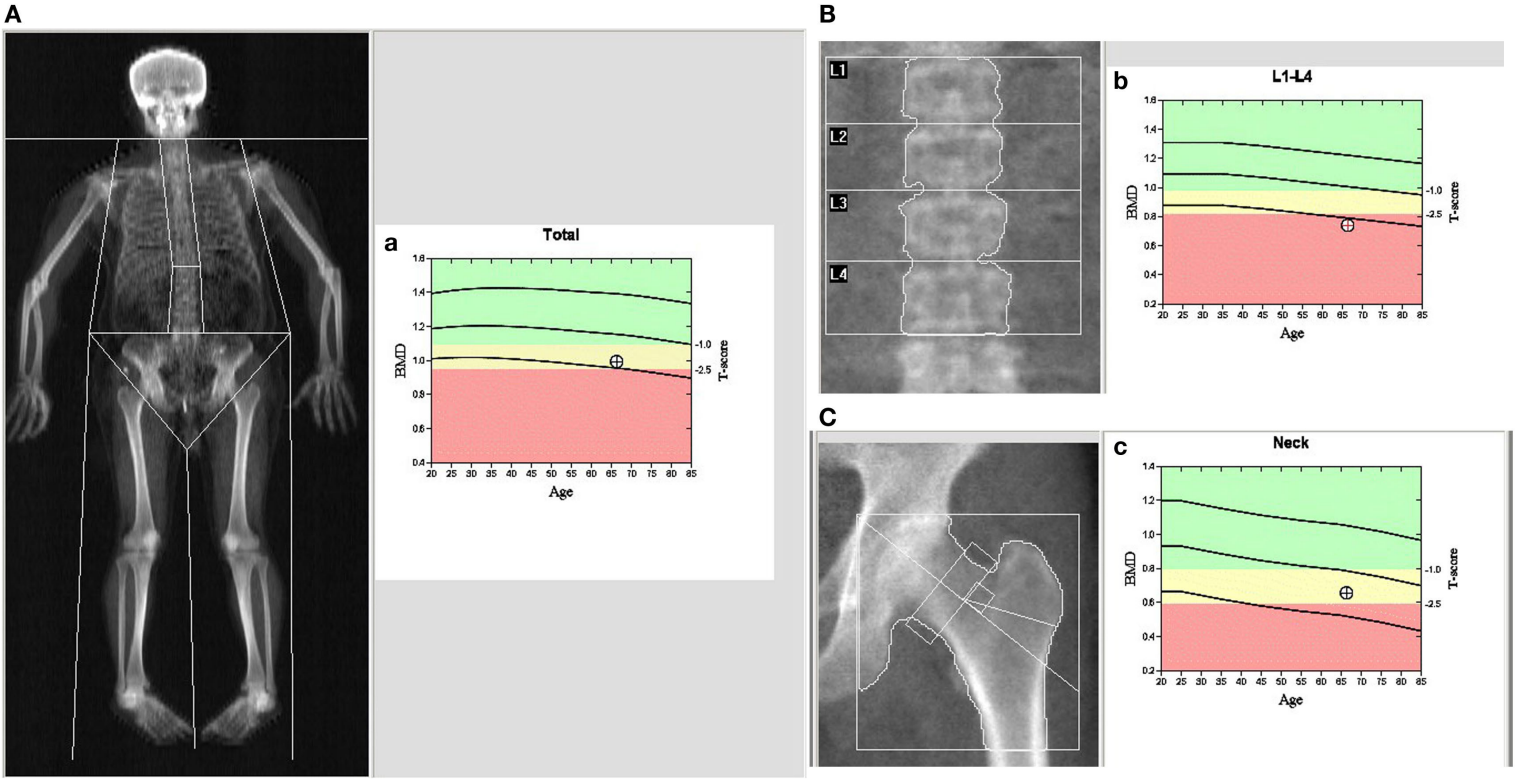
Dual-energy X-ray absorptiometry (DXA) imaging of BMD in a male PD patient: (A) the whole body, (B) spine, and (C) left hip. (a–c) The patients’ BMD vs. a male Chinese control of the whole body (a), spine (b), and left hip (c). In (a–c), the first cure line stands for baseline in the different ages; the second cure line stands for the boundary between normal BMD and osteopenia; the third cure line stands for the boundary between osteopenia and osteoporosis, and the round dot stands for the patients’ BMD.
